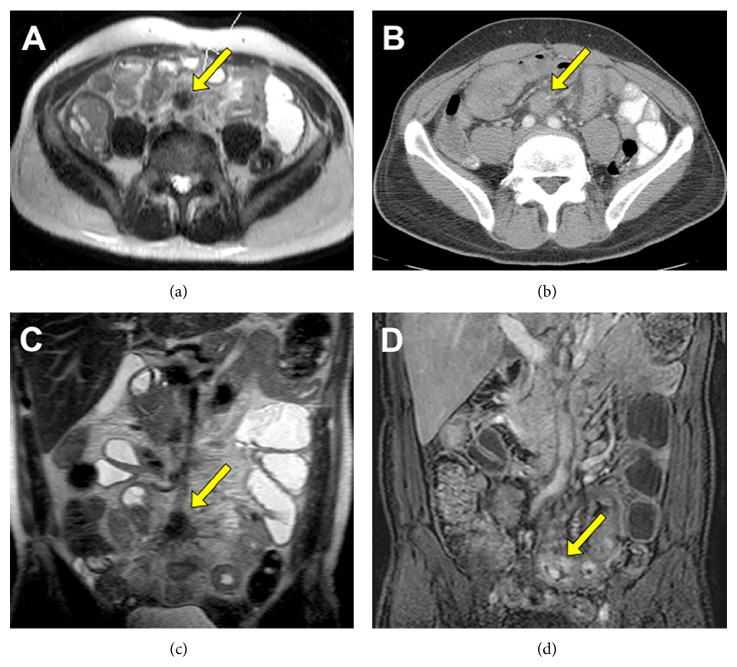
Axial MRE image showing mass in the small bowel mesentery and an enlarged lymph node (a) compared to an axial CT scan that only shows bowel wall thickening (b). Coronal MRE images with carcinoid tumor and an enlarged lymph node in the mesentery (c) and mass in the bowel wall (d).
